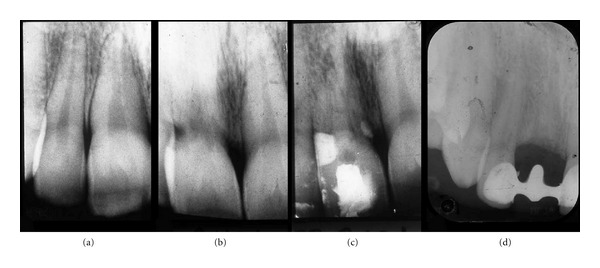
Patient 1: radiographs of the right maxillary central incisor region. (a) 1994. Seemingly normal periodontal conditions in the central incisor region. (b) 1997. Severe cervical resorptions have appeared in the central incisor. (c) August 1999. Severe resorption of the root of the maxillary central incisor. The condition resulted in extraction of the central incisor. (d) December 1999. The maxillary central incisor has been extracted and the alveolar bone level is normal.
